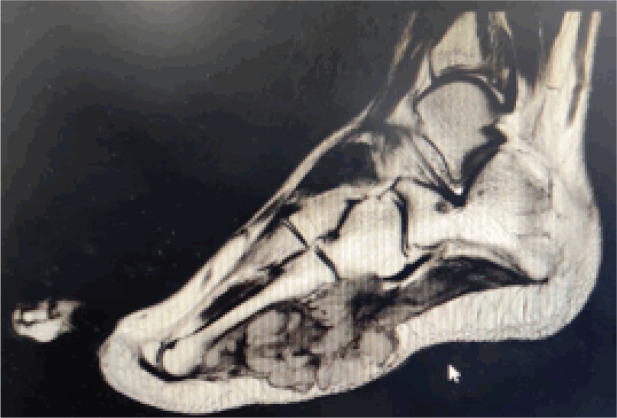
MRI image of right foot (T1W post contrast). Lobulated soft tissue mass with poorly defined margins in the lateral plantar aspect of right foot measuring approx. 4.5 × 7.5 × 3.8 cm (CC × AP × TR). Non- enhancing regions likely representing cystic/necrotic changes. Insinuating between the 4th and 5th metatarsals and involving the intrinsic muscles and abutting the lateral aspect of flexor digitorum longus/brevis muscles and tendons. Also involving the subcutaneous tissue on the plantar aspect of the foot. No definite evidence of signal abnormality or destructive changes in the bones.
